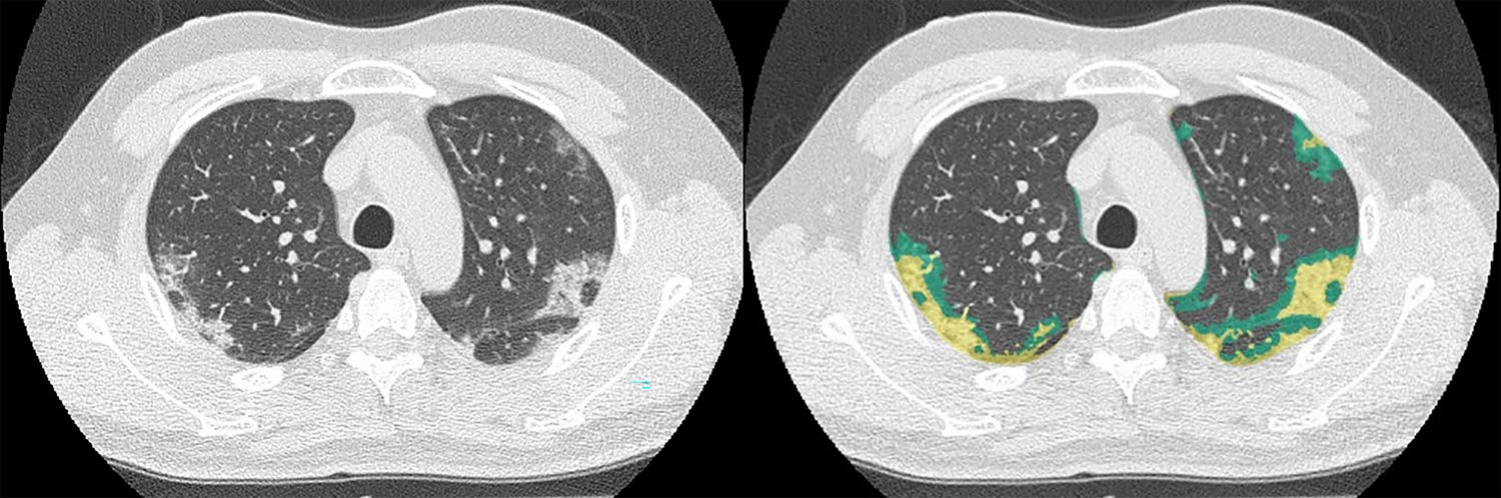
46-year-old male patient diagnosed as COVID-19 pneumonia and as positive on RT-PCR. (L to R: thin-section CT to CAST result) On thin-section CT, ground-glass opacities and reticulation classified as crazy-paving pattern were observed in the peripheral lung in both lungs. CAST shows ground-glass opacities as green and reticulation classified as crazy-paving pattern as yellow in both lungs. The PCR test also identified this case as “positive”. All chest radiologists and the CAST software accurately evaluated this case as “positive case”, and it was therefore classified as true-positive in this study
